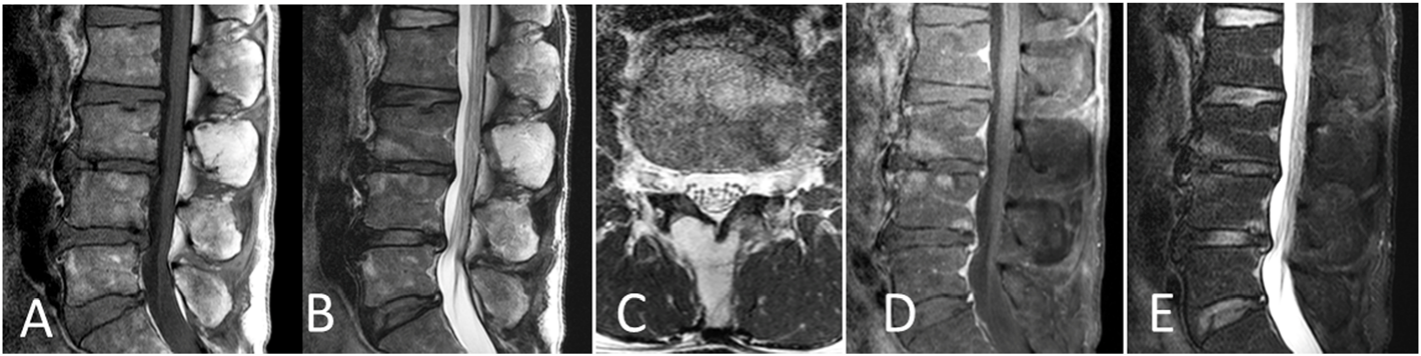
Magnetic resonance imaging at the L3 vertebral arch and spinous process. (A) Sagittal T1-weighted image; (B) Sagittal T2-weighted image; (C) Axial T2-weighted image; (D) Sagittal fat suppression image; (E) Sagittal T1-weighted gadolinium contrast (+) image.
